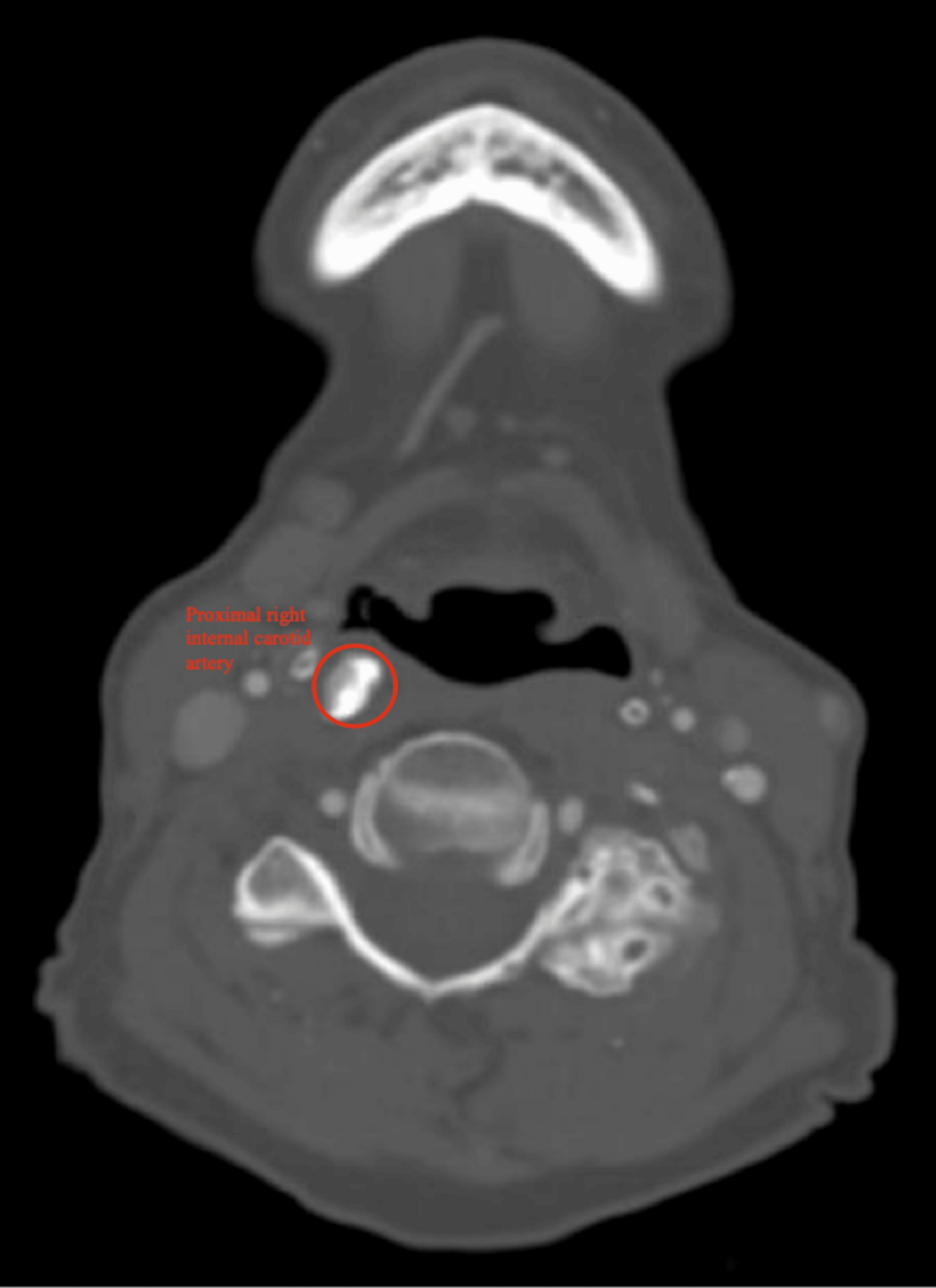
CT angiography of the neck showing severe stenosis of the proximal right internal carotid artery (circled in red) CT, computed tomography
